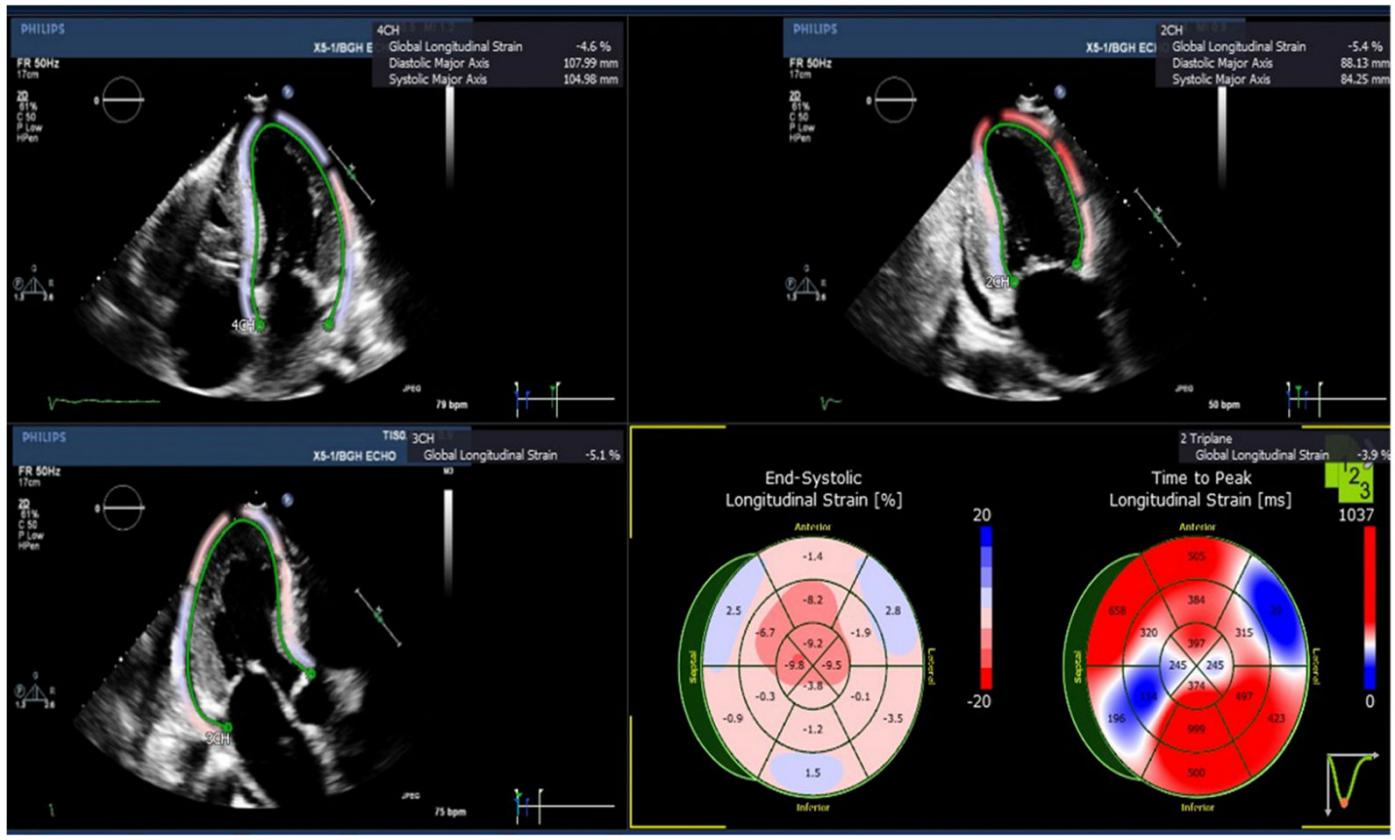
Left ventricular (LV) longitudinal strain imaging of cardiac amyloidosis using speckle tracking echocardiography. The basal segments are noted to exhibit dyskinetic motion, as observed by the outward movement of the basal inferior, basal anteroseptal and basal anterolateral segments. The apical segments are more contractile, but still relatively hypokinetic.
